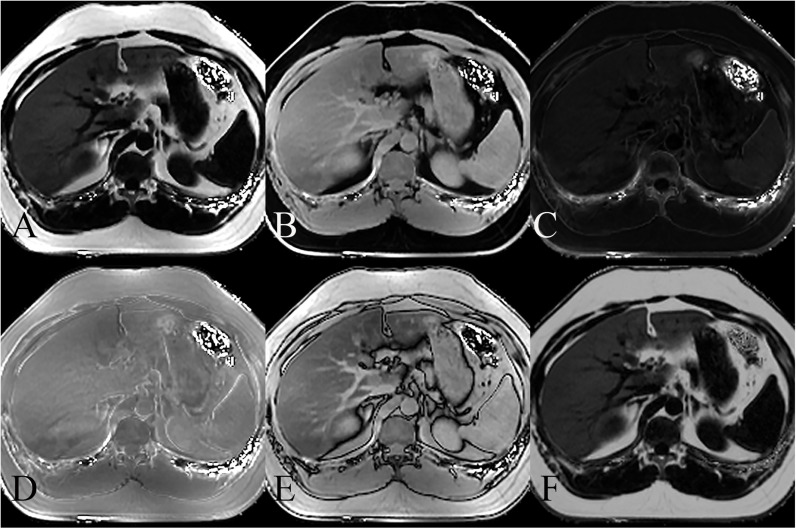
MRI images displaying various sequences used for MRI-PDFF measurement of NAFLD. (A) Fat image, showing the fat distribution in the liver. (B) Water image, highlighting the water content in the liver. (C) R2* map, representing the tissue relaxation rate. (D) In-phase water-fat imaging, where water and fat signals are in phase. (E) Out-of-phase water-fat imaging, showing water and fat signals out of phase. (F) Fat fraction map, quantifying the liver fat content.
